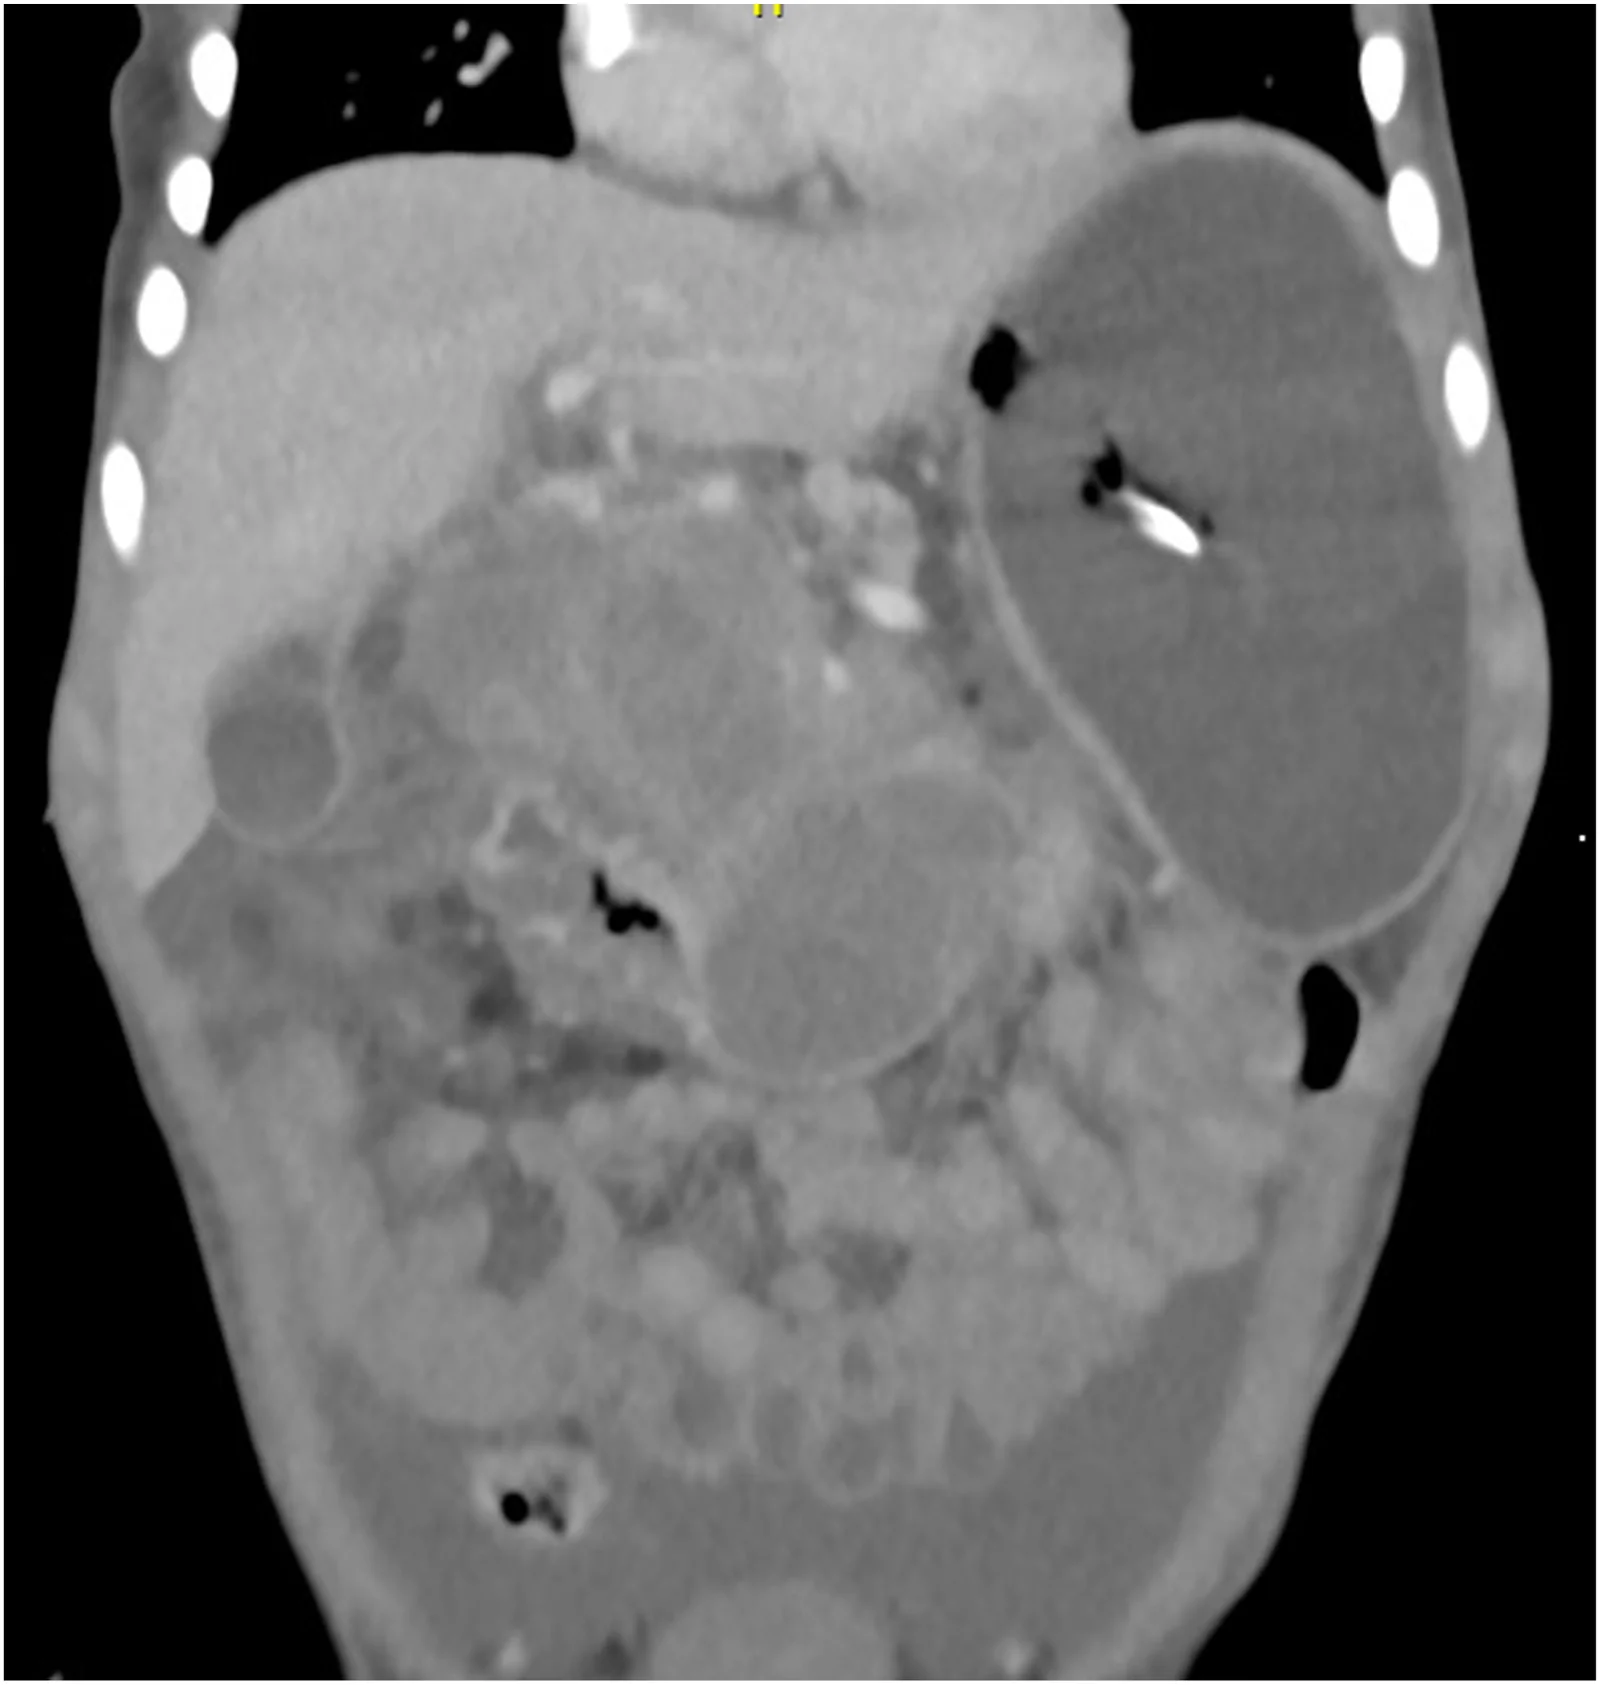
CT image showing gastric outlet obstruction secondary to the compression and invasion of the third part of the duodenum by the neuroblastoma.
